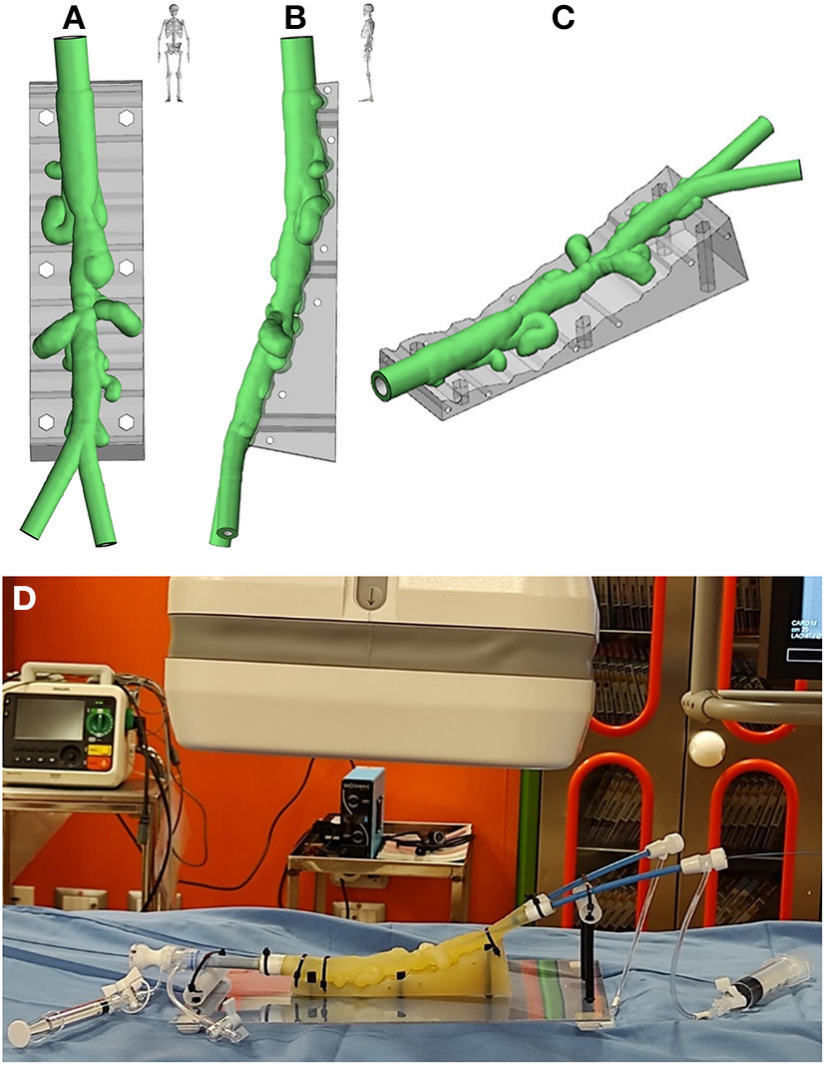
Antero-posterior (A), latero-lateral (B), and isometric (C) views of the virtual rendering of the 3D-printed model, replicated from patient-specific CT imaging; (D) 3D-printed model mounted on the *in vitro* planning platform.
